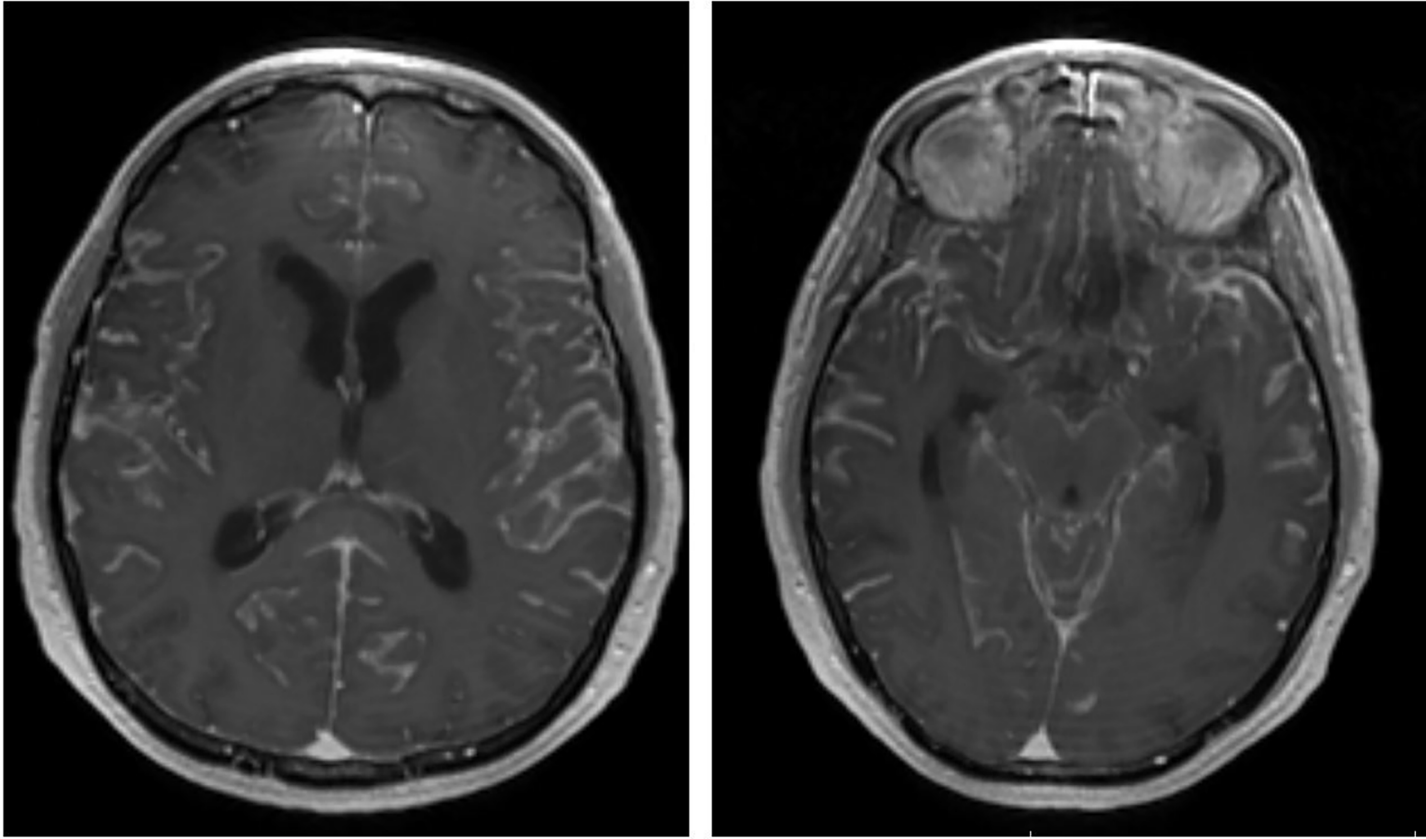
Representative post-contrast brain MRI (axial) showing diffuse LMD with enhancement within the cerebral sulci.
